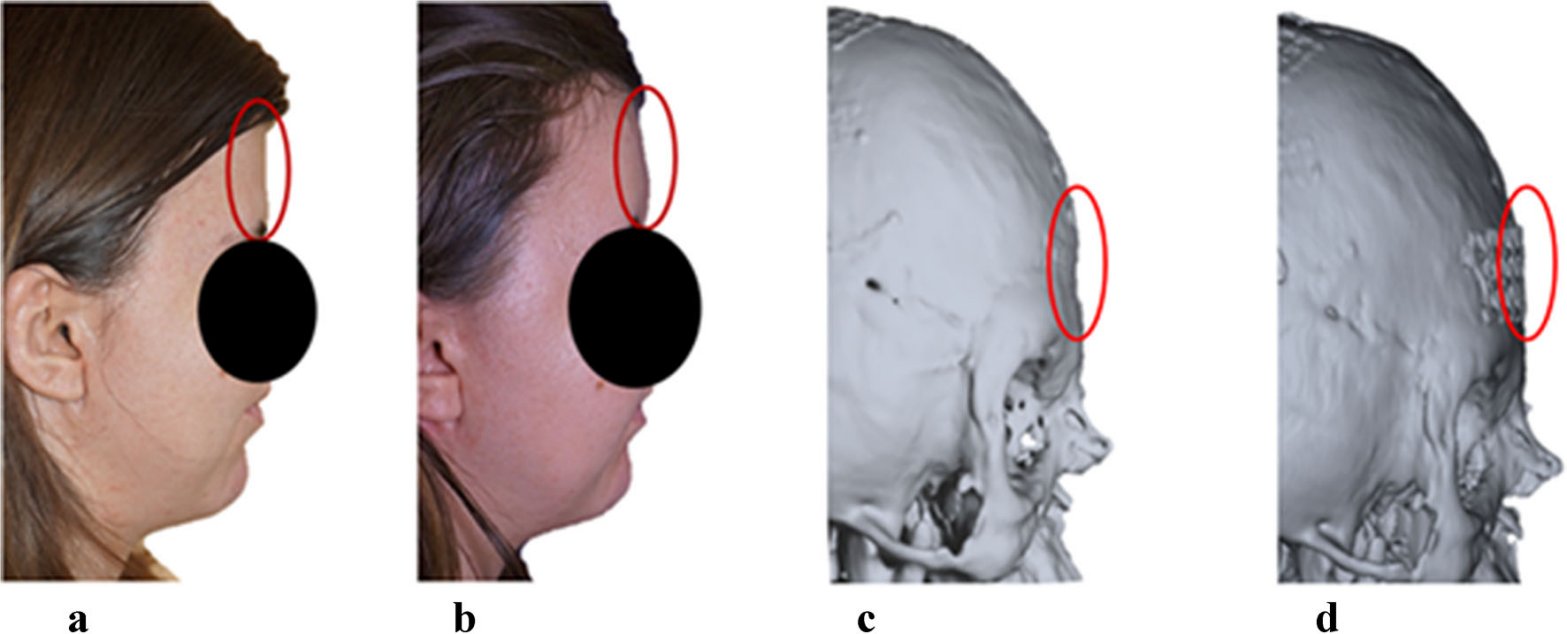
Pre (a) and post (b) surgical outcomes. The red circles highlight the affected region. Pre- (c) and post-(d) operative CT. Only the titanium mesh is visible because of the radiolucent nature of polyethylene
